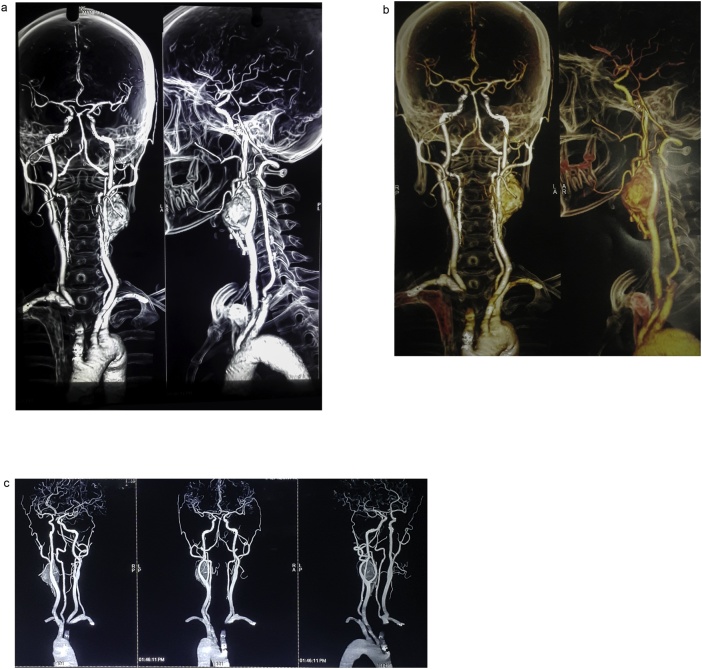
(a–c) CT Angiogram of neck vessels showing heterogenously enhancing lesion at the left carotid bifurcation with splaying of left internal and external carotid arteries.
